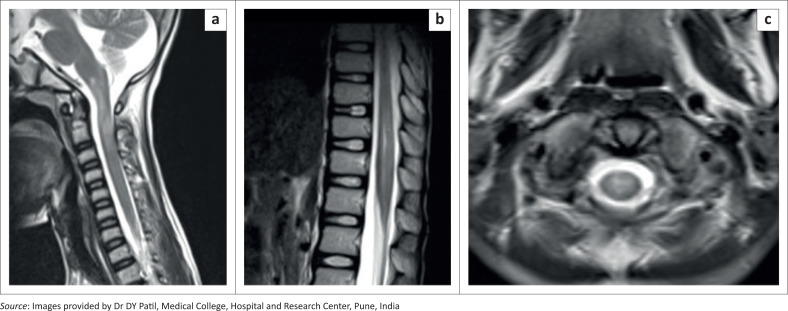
(a–c) [Case 2]: MRI screening of the spine in a 10-year-old male demonstrating T2 hyperintense signal in the inferior part of the medulla, cervicomedullary junction, upper cervical spinal cord, lower dorsal cord and conus medullaris.
